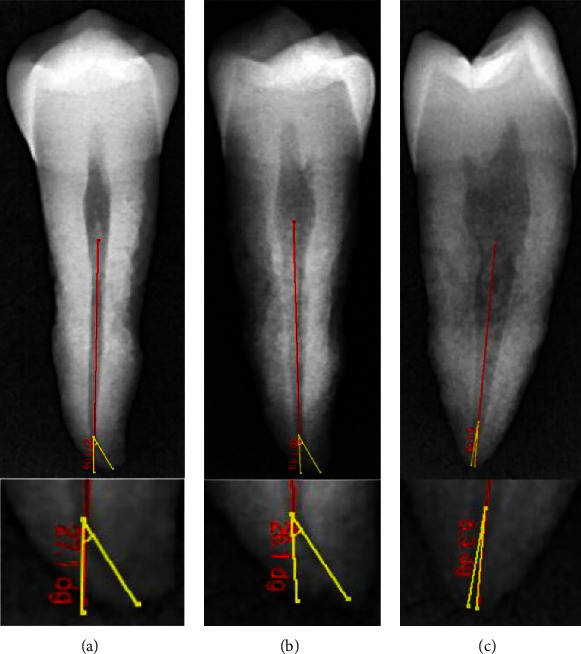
Representative digital radiographic images demonstrating a canal curvature angle measurement for a tooth without root angulation using a Schneider method. (a) 0° angle buccolingual view. (b) 30° angle horizontal parallax view. (c) 0° angle proximal view.
